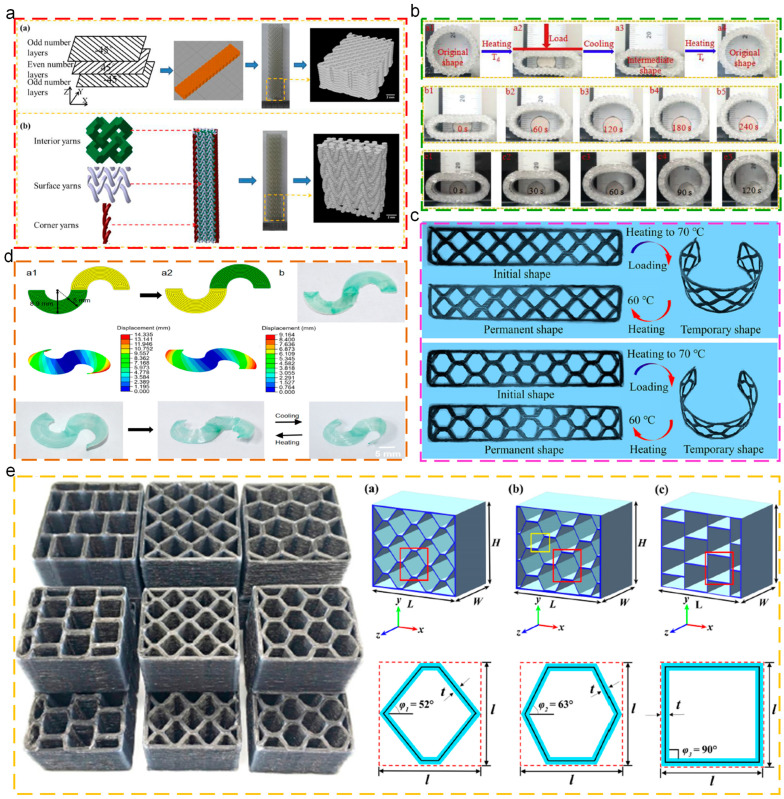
Applications of thermoplastic SMPs in engineering structures: (a) microstructural design and μ-CT imaging of 4D-printed preforms [149]; (b) shape memory cycle of 4D-printed circular braided tube [150]; (c) initial shapes, temporary shapes, and permanent shapes of 3D-printed CFRCTCSs [151]; (d) bidirectional bending reversible deformation of S-shaped semicircular ring model, which was filled with concentric circular printing path [152]; (e) prepared samples and geometric configurations of proposed honeycomb structures with various cells [153].
